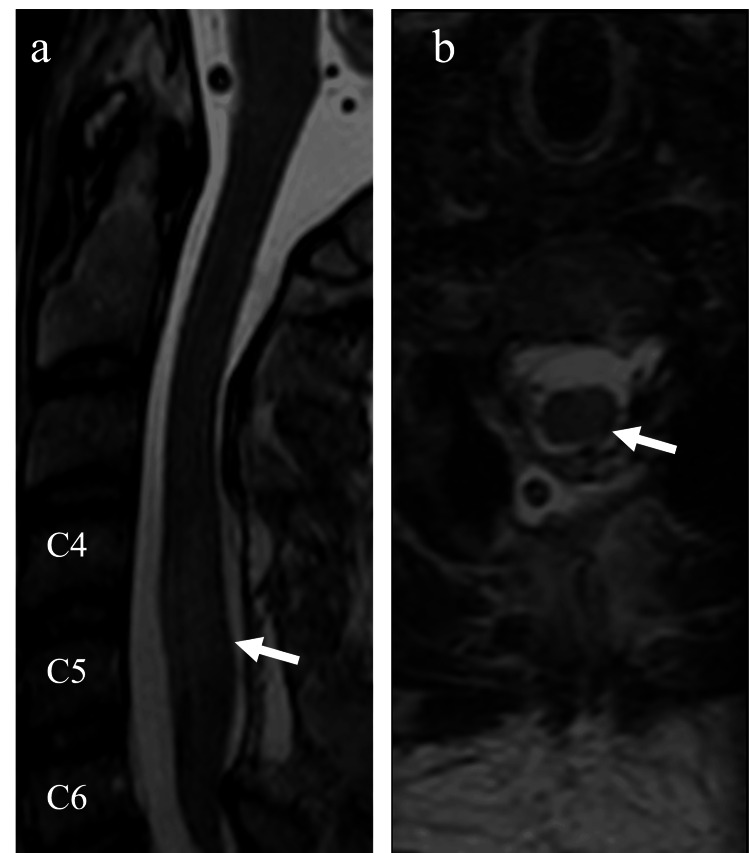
Postoperative MRI Sagittal T2-weighted image (a) and axial T2-weighted image (b) showing a sufficient decompression of the spinal canal with spinal cord edema (arrow) from C4 to C6 level. There is no residual hemorrhage visible (a, b).
